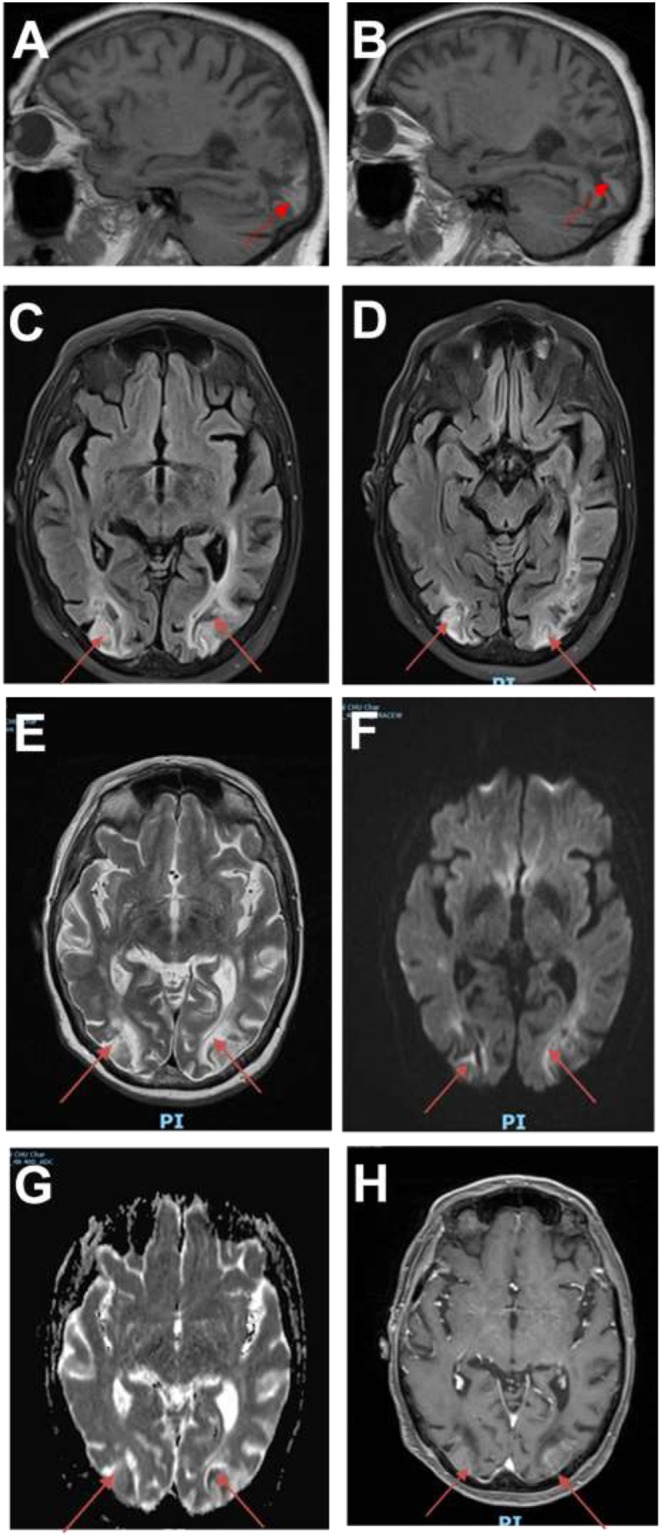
Follow-up images. (A,B) Sagittal T1 MRI showing hyperintensities in both occipital lobes (red arrows). (C,D) MRI fluid attenuation inversion recovery (FLAIR) images showing persistent ischemic-like lesions in the occipital lobes. (E) T2-weighted images showing hyperintense lesions in the occipital lobes. (F) Diffusion images demonstrating restriction in the occipital lobes. (G) MRI apparent diffusion coefficient (ADC) images showing residual restriction in the occipital lobes. (H) MRI angiographic sequences confirming persistent breakdown of BBB in the occipital lobes.
